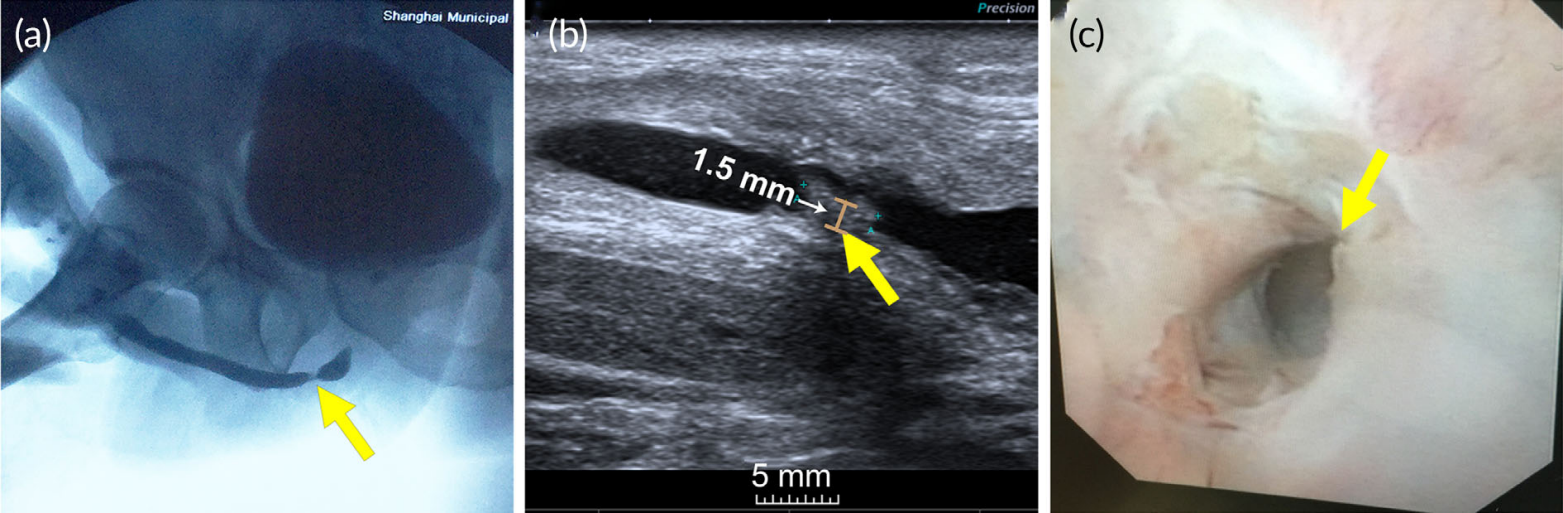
Representative (a) urethrography, (b) urethral ultrasonography, and (c) urethroscopy results of a patient with a bulbar urethral stricture. The yellow arrow indicates the segment of urethral strictures, the brown line indicates the maximum thickness of the urethral scar
